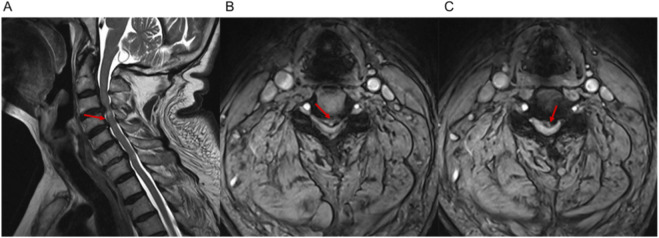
Preoperative cervical spine MRI showing disc protrusions and stenosis at C3/4 and C4/5 levels. (A) Sagittal view showing signal changes in the spinal cord at the C4 level, with significant disc protrusions and stenosis at C3/4 and C4/5 levels. (B) Axial view of the C3/4 intervertebral disc showing disc protrusion and canal narrowing. (C) Axial view of the C4/5 intervertebral disc showing similar findings of disc protrusion and stenosis.
